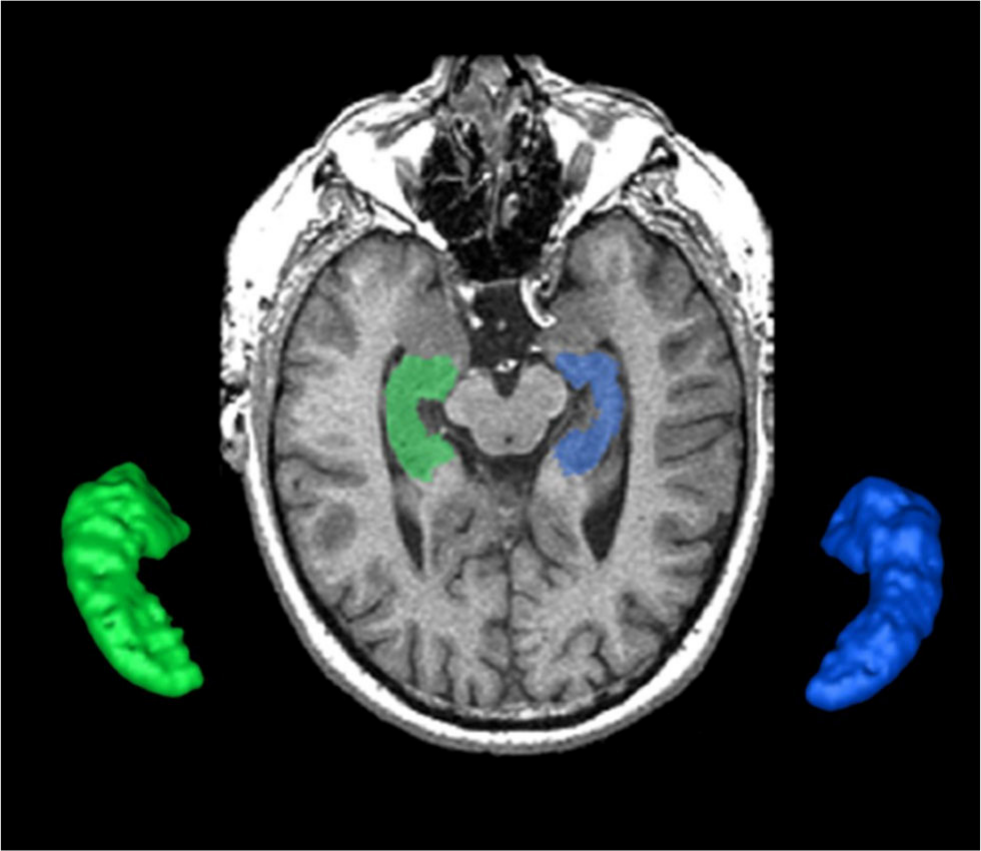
The SunnyBrook Hippocampal Volumetric (SBHV) segmentation showing left (BLUE) and right (GREEN) hippocampi overlayed on an axial T1 MRI and extracted as 3-D surface volume renderings. Note images are in radiological convention.
